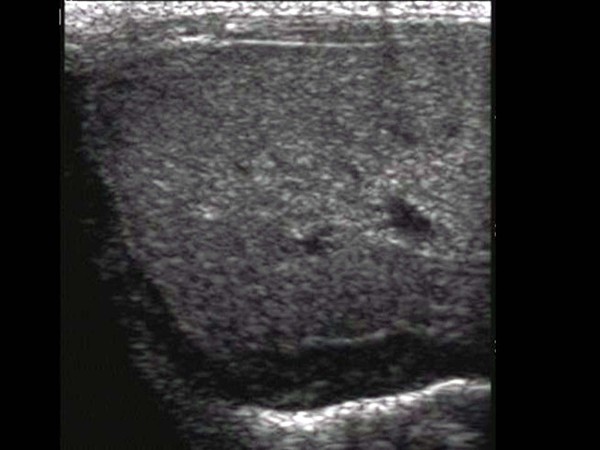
A follow-up sonogram obtained after seven days reveals a markedly improved cystic lesion in the lateral portion of the liver.
